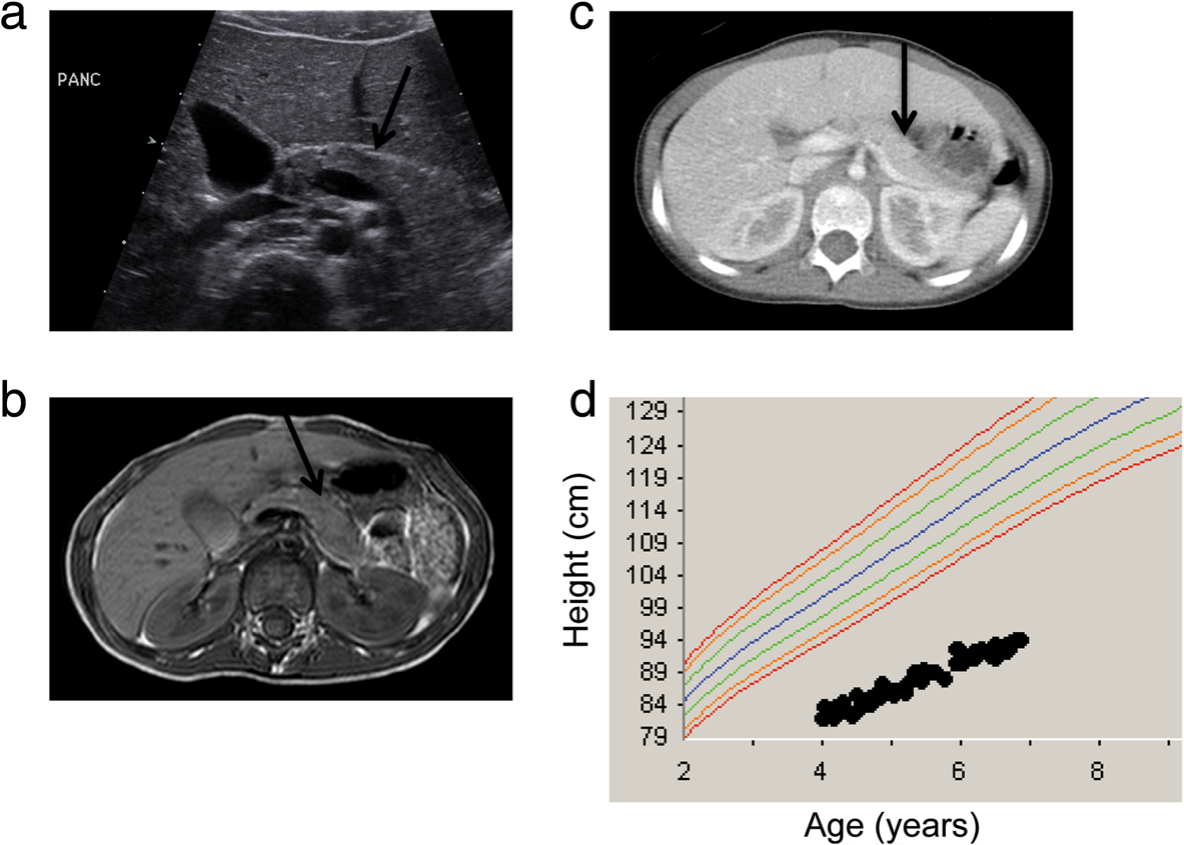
Clinical features of SDS proband. a. Transverse abdominal ultrasound image obtained at 5 years 11 months of age revealing normal size, contour, and echogenicity of the pancreas, indicated by arrow. b. Axial conventional spin echo T1-weighted MR image obtained at 5 years 11 months of age showing normal signal intensity of the pancreatic parenchyma with no evidence of atrophy or fatty infiltration of the pancreas, indicated by arrow. c. Axial contrast-enhanced CT image obtained at 6 years 5 months of age demonstrating normal attenuation of the pancreatic parenchyma with no evidence of atrophy or fatty infiltration of the pancreas, indicated by arrow. d. The proband’s growth curve showing markedly short stature. The bottom-most curve normal curve represents the 5th percentile for girls.
